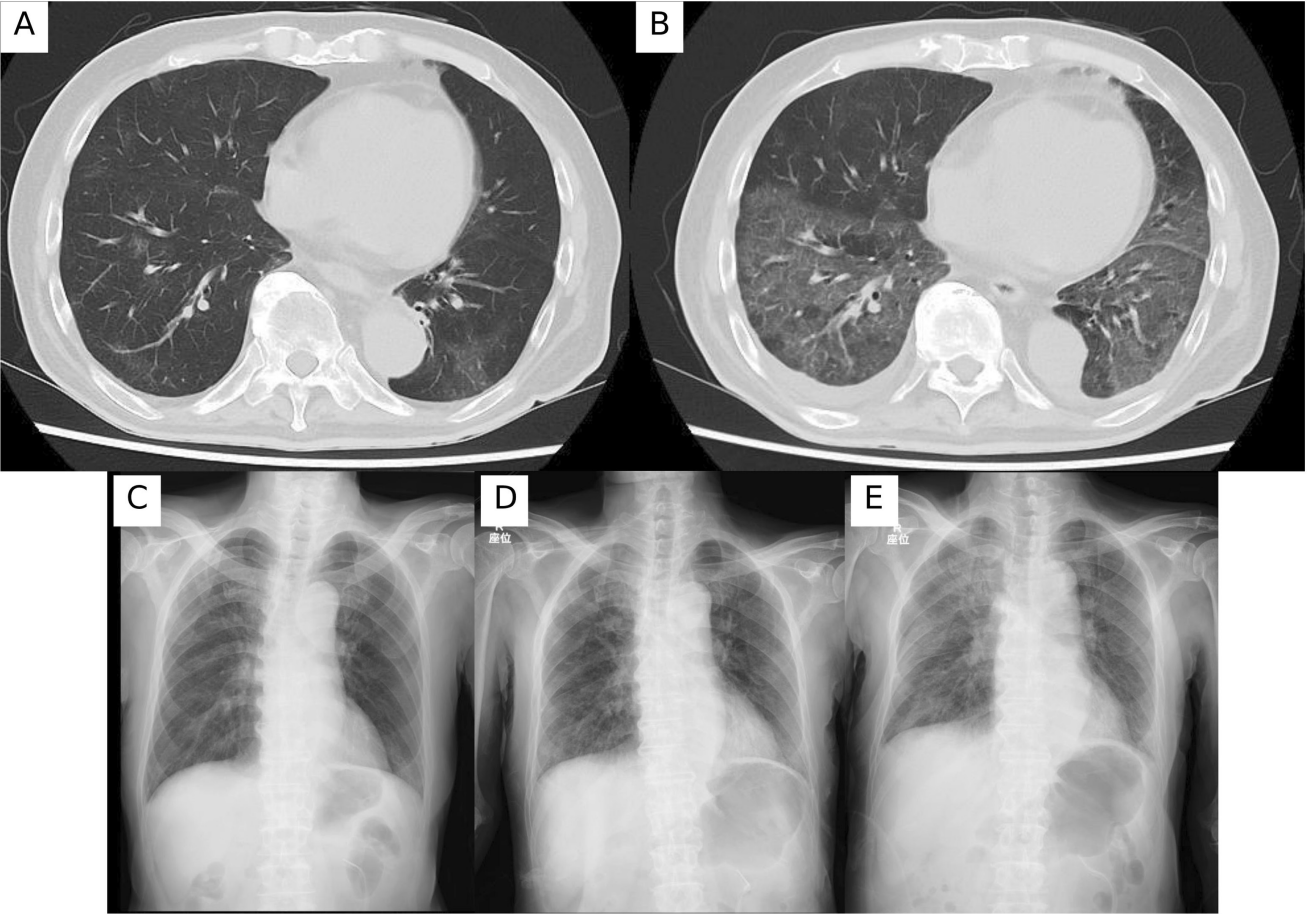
Serial thoracic imaging demonstrating progressive Pneumocystis jirovecii pneumonia. (A, B) Axial chest CT scans on hospital days 1 and 7 showing progressive bilateral ground‐glass opacities and interlobular septal thickening (arrows). (C–E) Upright anteroposterior chest radiographs on hospital days 1, 5 and 9 demonstrating worsening diffuse reticular and ground‐glass opacities consistent with non‐HIV Pneumocystis jirovecii pneumonia.
